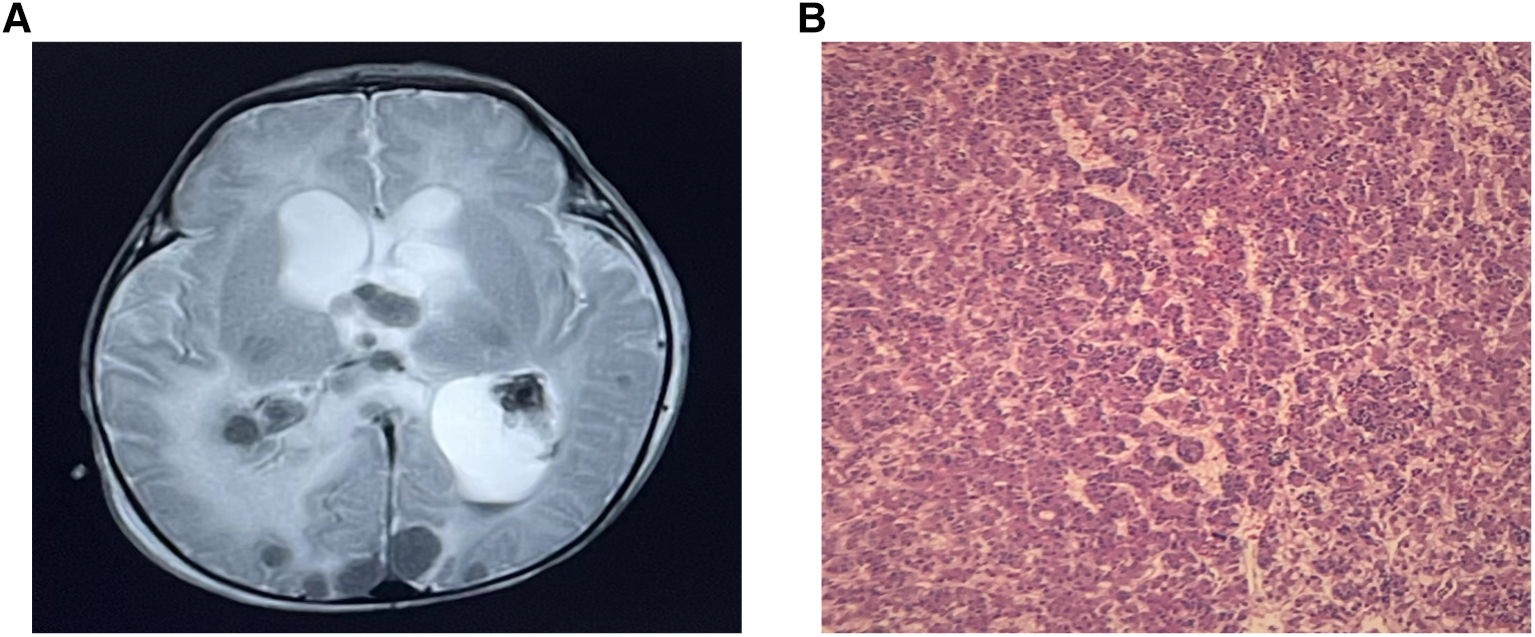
(A) Brain MRI demonstrated multiple intracranial tumors. (B) The pathology revealed an epithelial-type hepatoblastoma.
